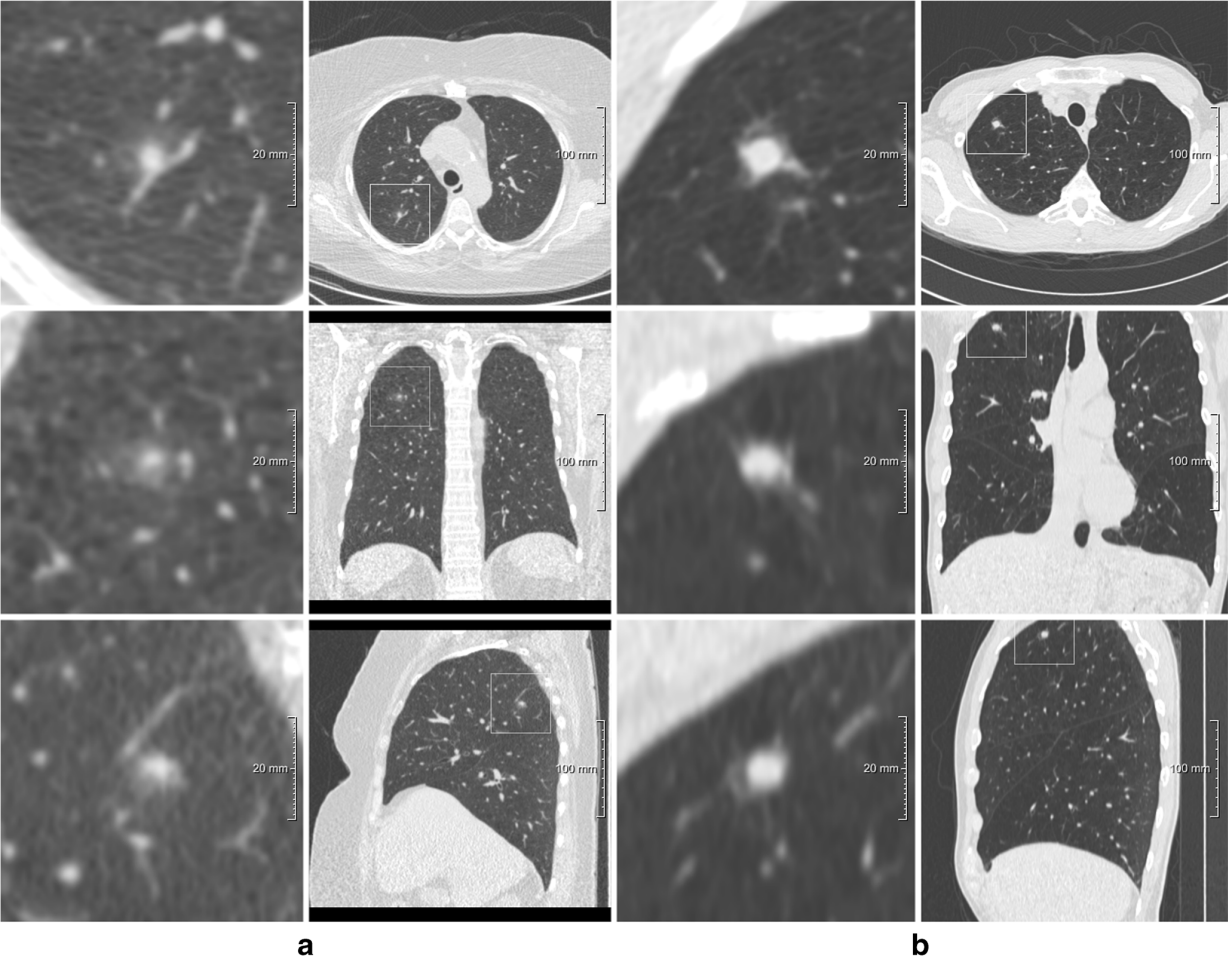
Two examples of risk-dominant nodules characterised differently by the seven observers which led to Lung-RADS classification differences. Each example shows a nodule displayed in magnified view (left column, field of view of 60 × 60 mm) and normal view (right column). The three different rows show axial (top), coronal (middle) and sagittal (bottom) plane. a T1 CT scan with a nodule that was classified as Lung-RADS 2 by one observer (new small solid nodule), Lung-RADS 4A by one observer (new part-solid with solid component < 4 mm) and Lung-RADS 4B by five observers (new part-solid, with solid component > 4 mm). b T1 CT scan with a nodule that was classified as Lung-RADS category 4A or 4B by five observers (new solid nodule with a measured diameter ranging from 7 to 9.6 mm) and Lung-RADS category 4B by two observers (new part-solid nodule with a solid component > 4.0 mm)
